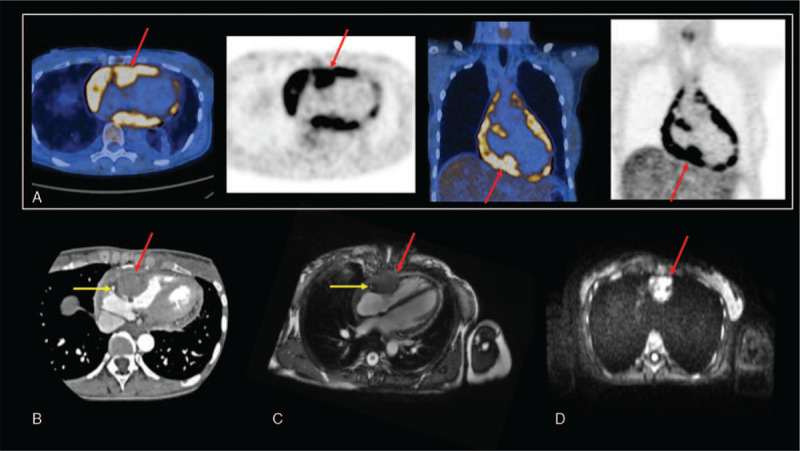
(A) Axial and coronal fused PET/CT and PET images of the thorax showing an intense and pathological FDG uptake in the basal posterolateral wall of the right ventricle (red arrow) and in the pericardium. (B) Cardiac CT scan image shows the cardiac metastasis infiltrating the basal posterolateral wall of RV (red arrow), in front of the right coronary artery (yellow arrow) with a thickening of the entire pericardium. Cardiac magnetic resonance imaging. (C) 2D-SSFP (FIESTA) image four-chamber view shows a 4.6 × 3.6 cm mass infiltrating the basal posterolateral wall of RV (red arrow), in front of the right coronary artery (yellow arrow), extended to the basal posterolateral wall of right atrium. (D) Diffusion-weighted imaging at *b* = 1000 s/mm^2^ view shows an area of increased signal corresponding with the mass of RV (red arrow).
